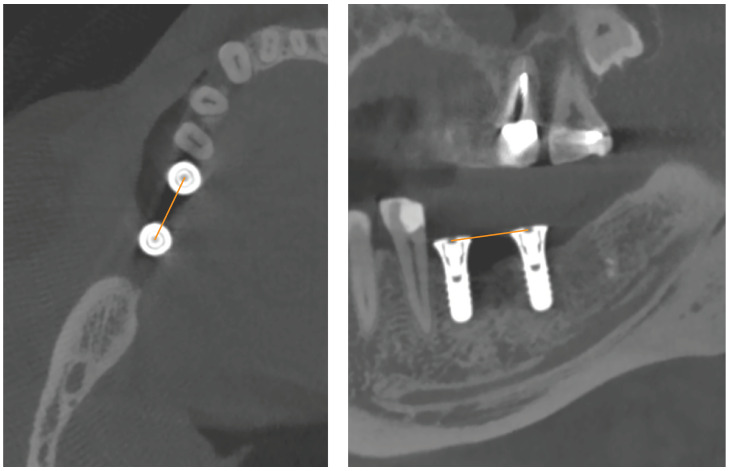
Horizontal linear measurements from the center of one implant healing cap to another (i.e., inter-implant distance) on CBCT axial and sagittal views.
